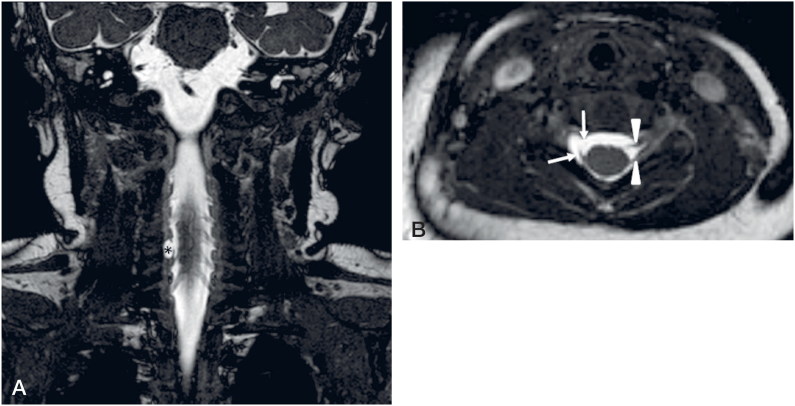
Coronal (A) and axial (B) BFFE MR images (0.5 mm) in a 3-month-old boy (patient 18) with brachial plexus birth injury on the right side. Total (both ventral and dorsal roots) avulsion of right C6 root with a PMC (asterisk): ventral root is avulsed from the cord (upper arrow), where a short stump of dorsal root is seen (lower arrow). Left ventral and dorsal C6 roots (arrowheads) are normal.
